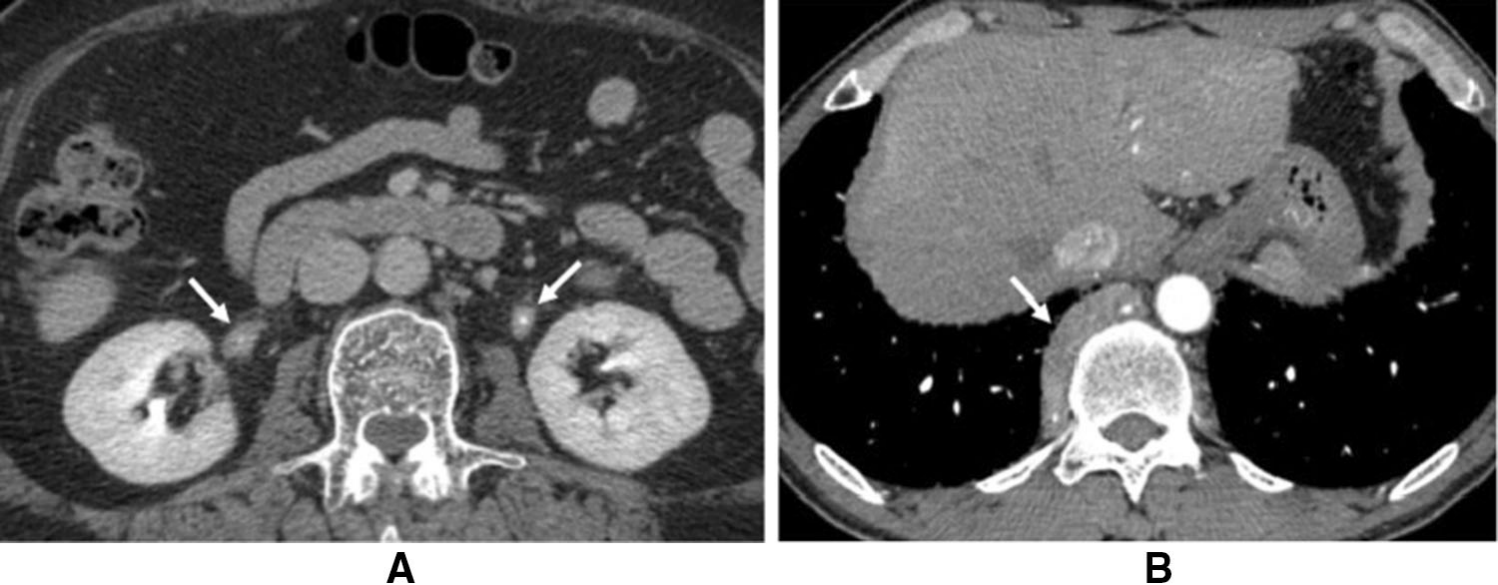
Enhanced CT images of AIP-related retroperitoneal fibrosis. A Enhanced CT (late phase) shows soft-tissue masses around both ureters (arrows). B Enhanced CT (arterial phase) reveals a soft-tissue mass anterior to the vertebra (arrow)
